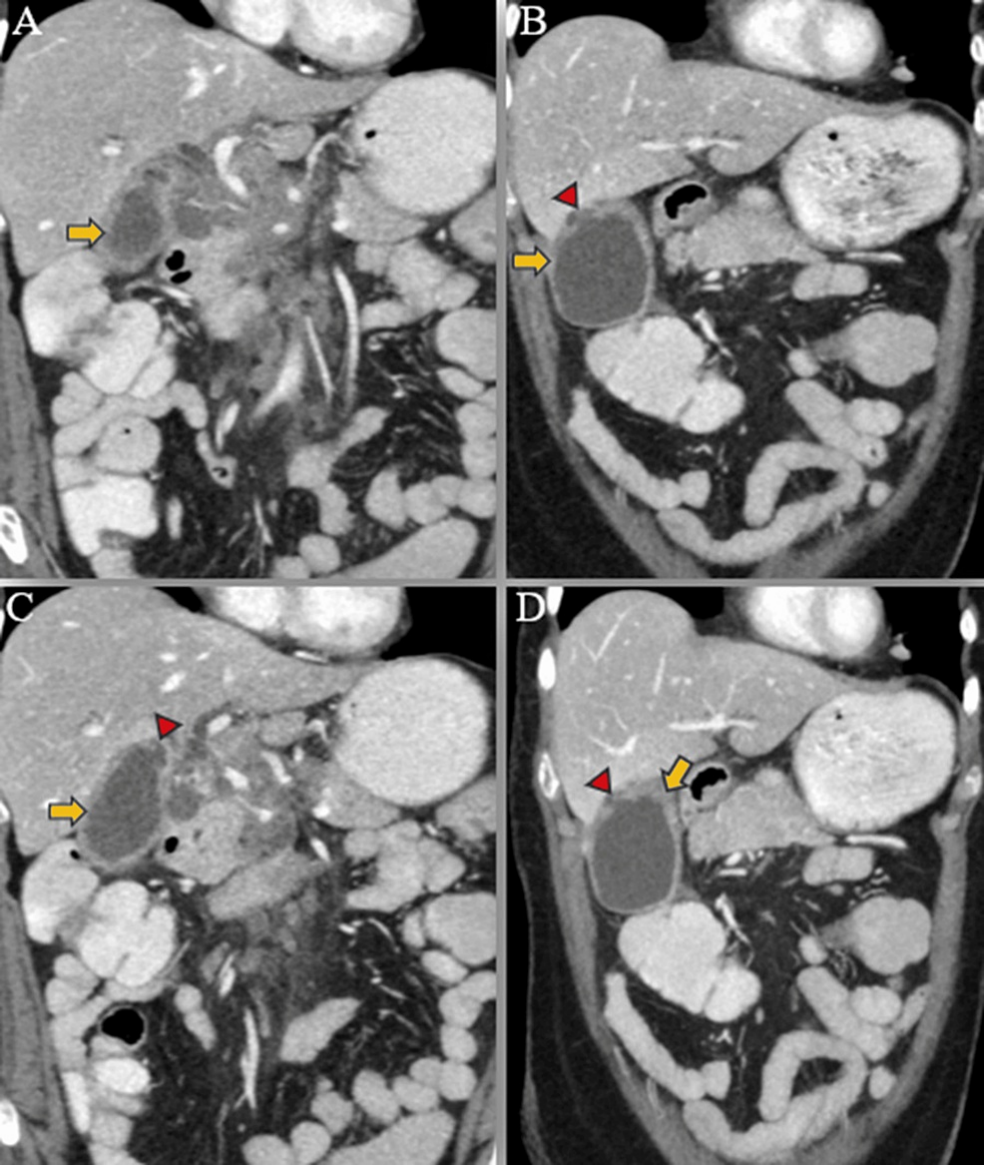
Post-contrast coronal reformatted CT images show a thickened wall of the gallbladder (yellow arrows) exhibiting post-contrast enhancement (A-D), with a defect (red arrowheads) observed in the wall of the body of the gallbladder, accompanied by a hypodense pericholecystic fluid collection (B-D).
